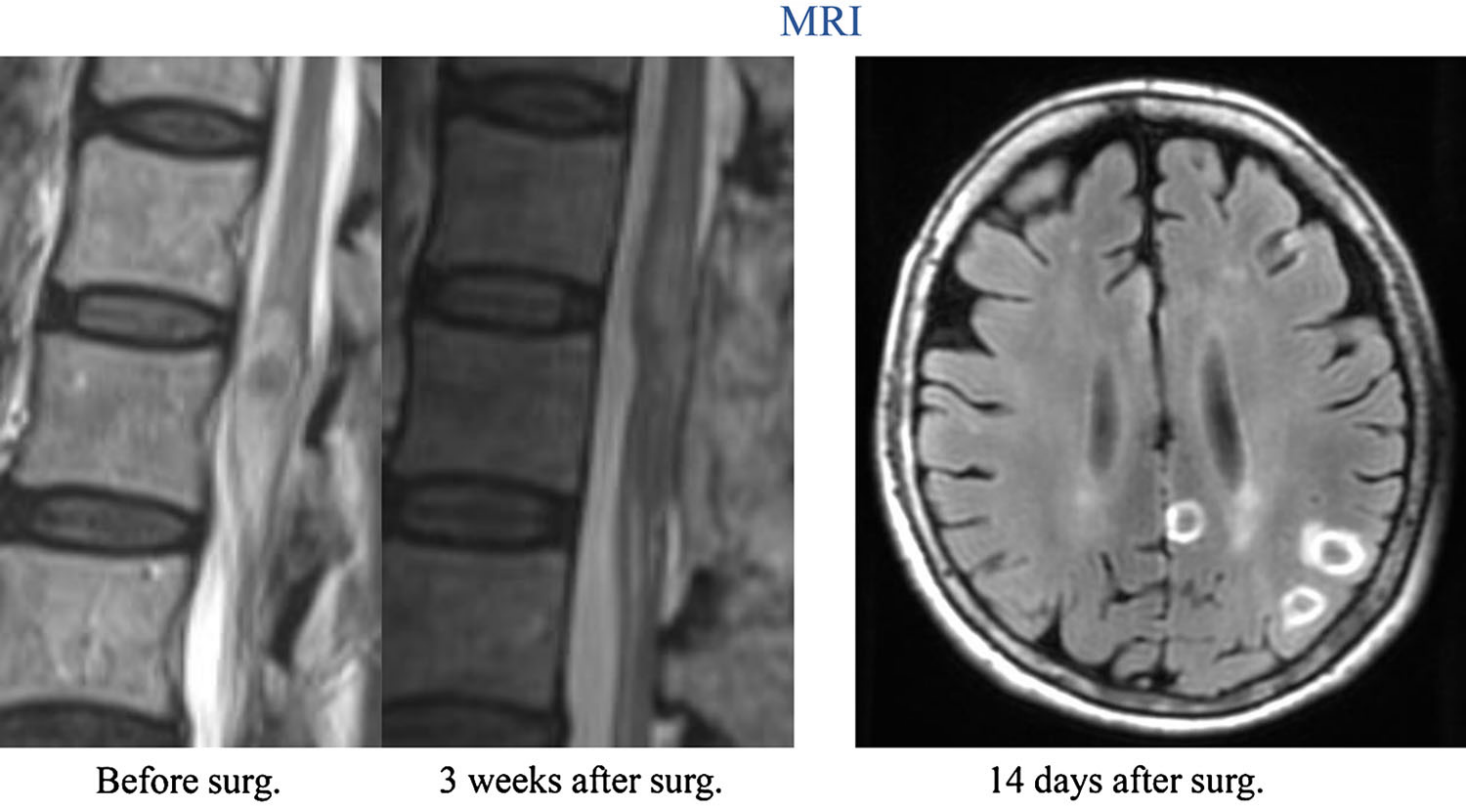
Postoperative MRI. *Left* after surgery, the abscess in the cauda equina was confirmed to have been completely resected. *Right* multiple brain abscesses were identified
